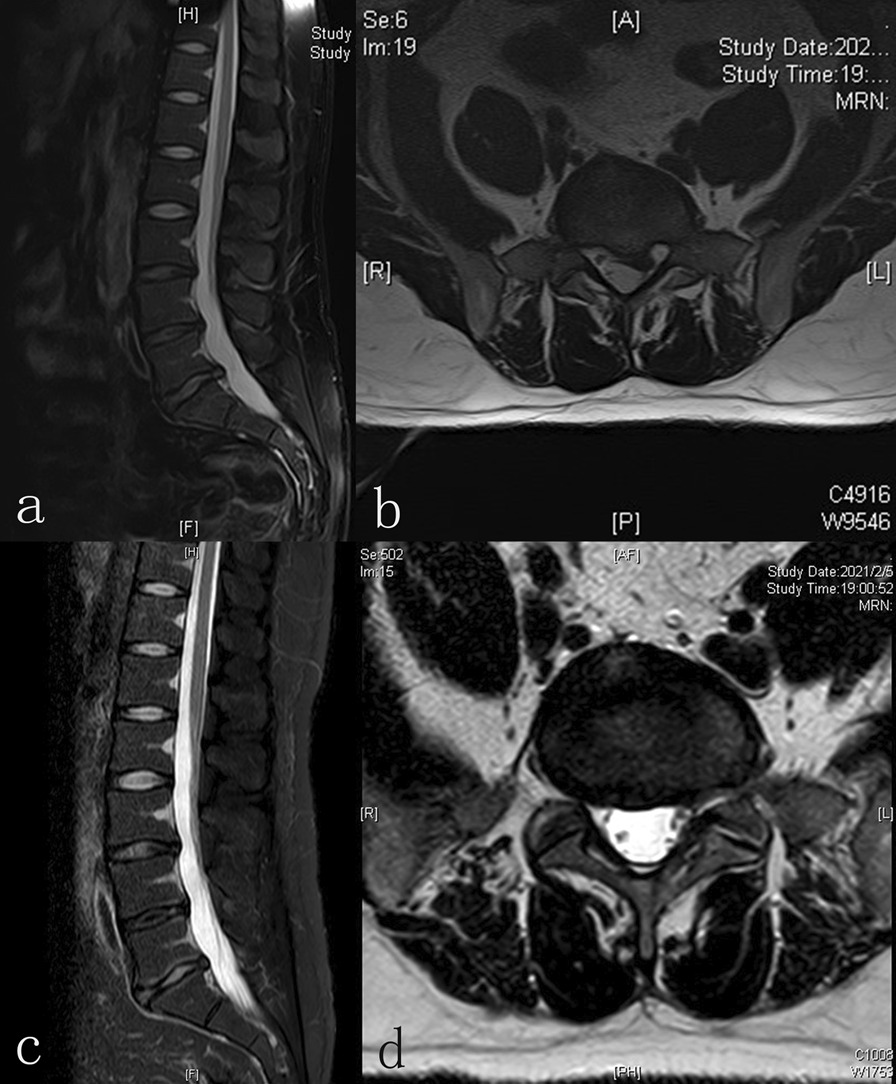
Representative magnetic resonance imaging (MRI). A patient was diagnosed with L5-S1 lumbar disc herniation (LDH). A sagittal and cross-sectional MRI revealed L5-S1 LDH pre-treatment (a, b). MRI sagittal and cross-sectional image of improvement after percutaneous endoscopic lumbar discectomy (PELD) combined with platelet-rich plasma (PRP) hydrogel injection at L5-S1 at 1 year post-treatment (c, d)
